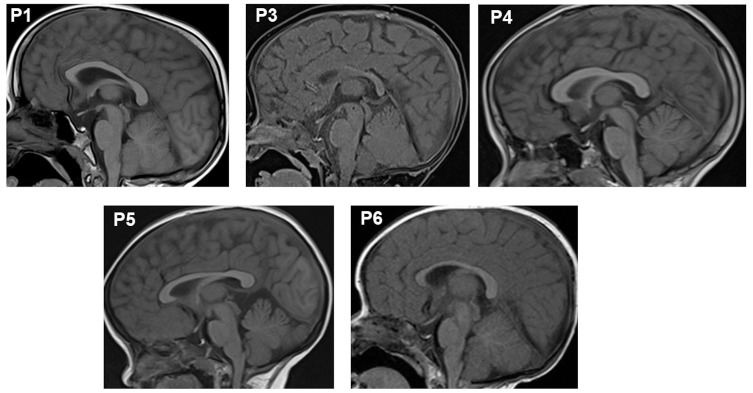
Magnetic resonance imaging (MRI) revealed the anterior hypoplasia in the pituitaries of patients.
